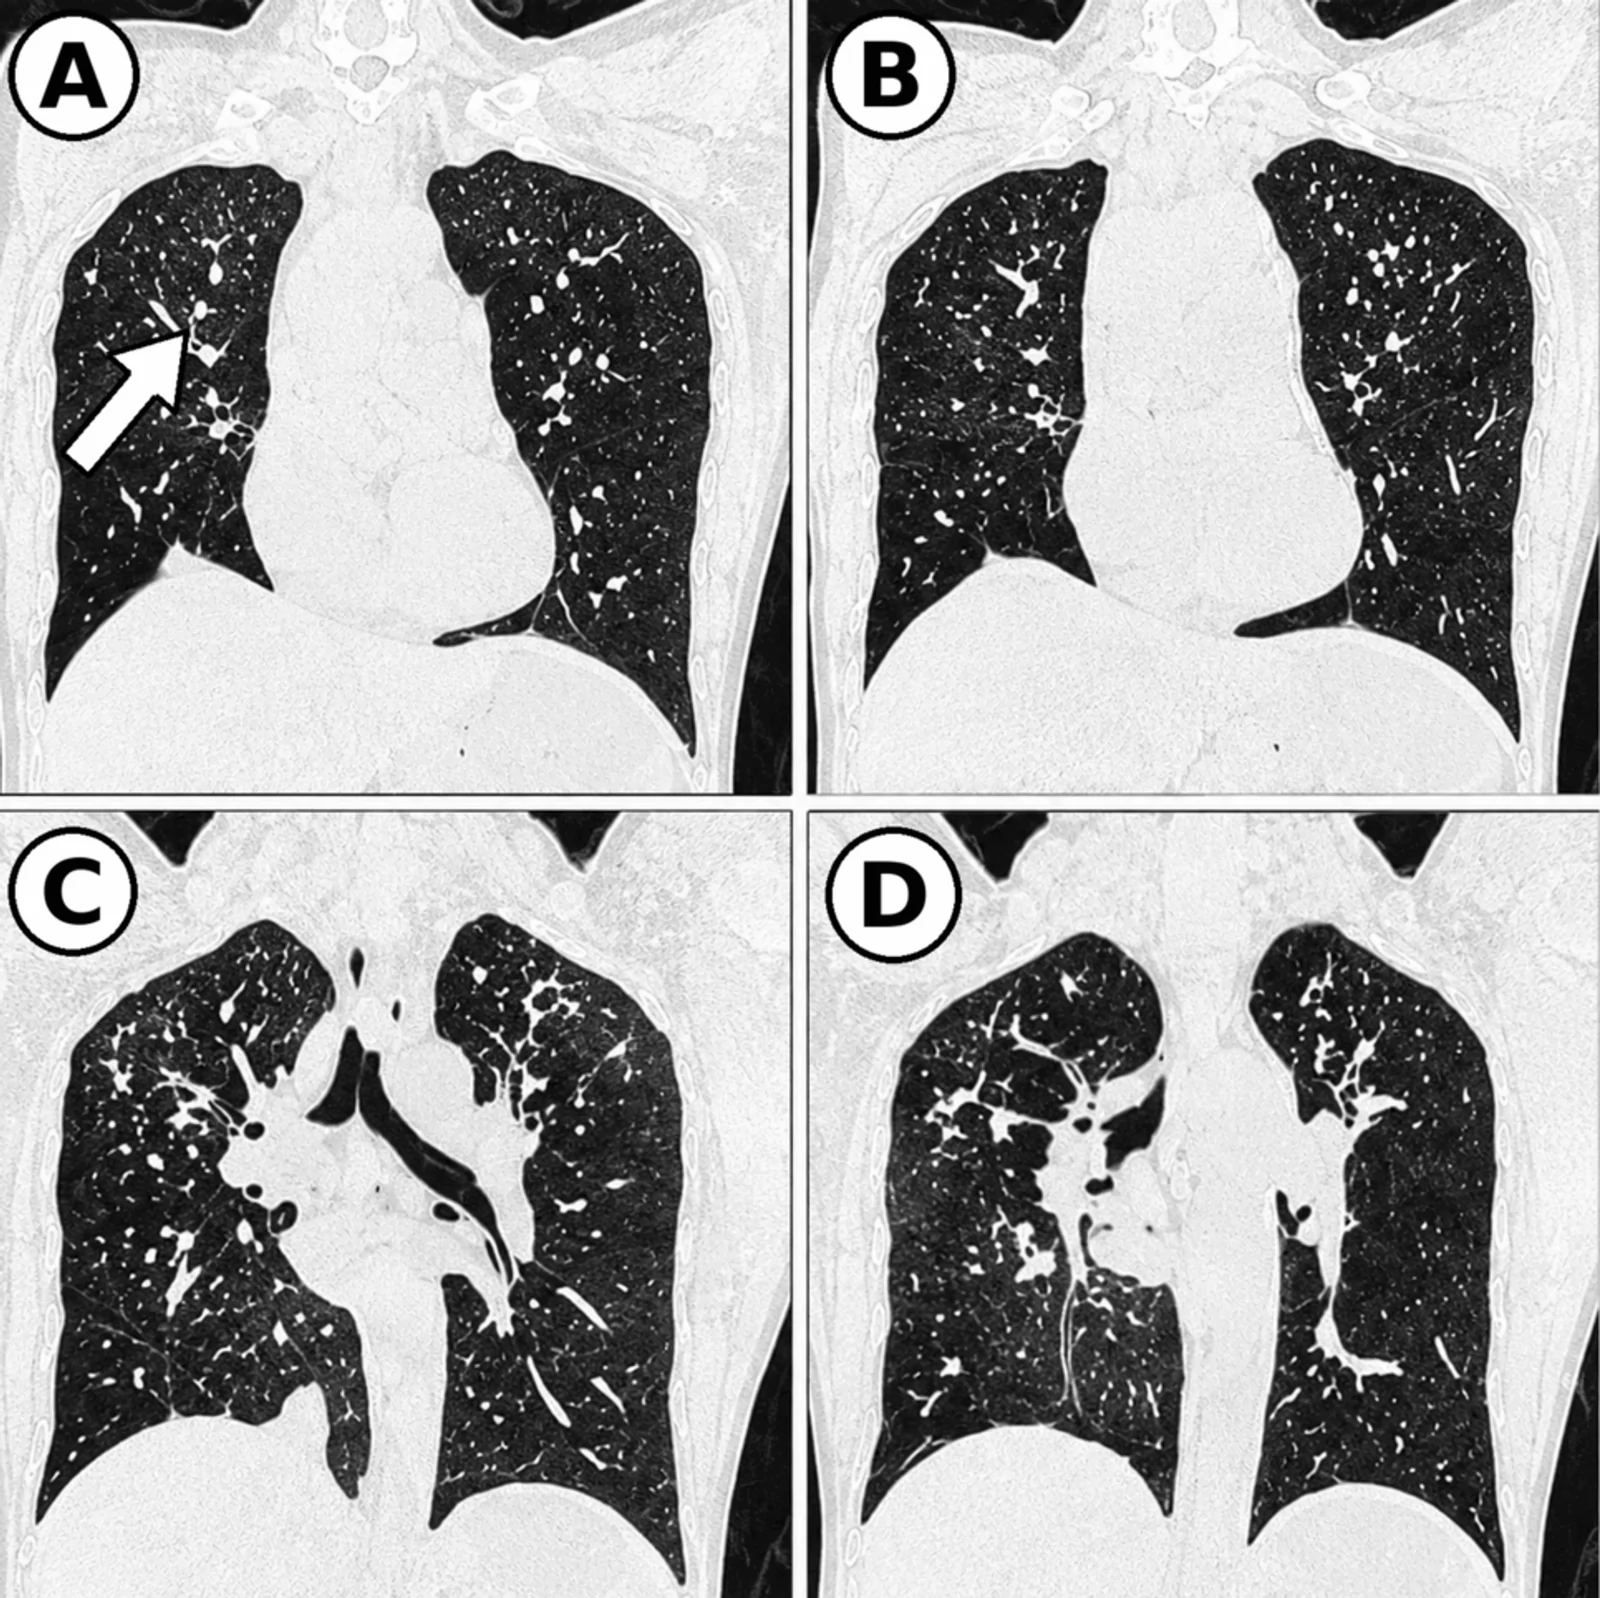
HRCT of the thorax (coronal view) showed multiple calcified pulmonary nodules Coronal HRCT image (A) showing calcified pulmonary nodules, with the solid white arrow indicating a representative calcified nodule; (B) at a subsequent level showing additional calcified pulmonary nodules; (C) showing further calcified nodules distributed through the lung parenchyma; and (D) showing the extent of calcified nodular involvement bilaterally HRCT: high-resolution computed tomography
